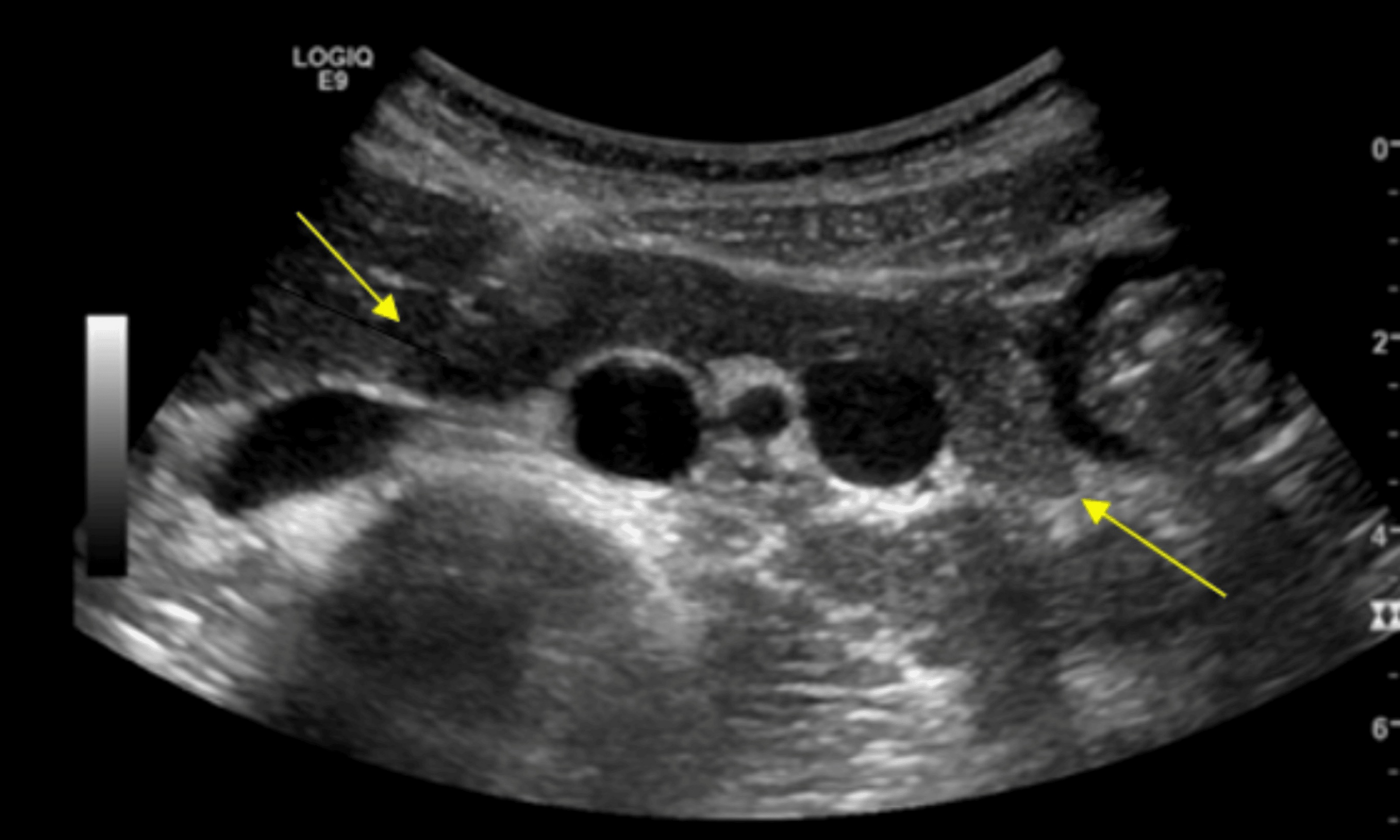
Ultrasound of the abdomen shows the pancreas is homogeneously enlarged with a mildly hypoechogenic appearance.
